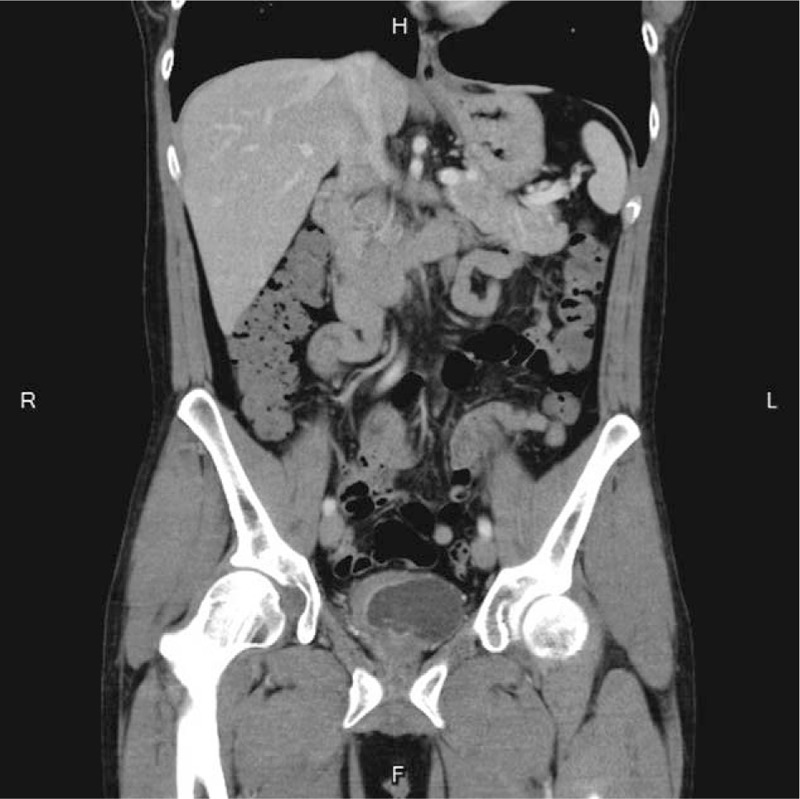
CT showed asymmetrical wall thickening (thickness of up to 1.2 cm) of the anterior aspect of the urinary bladder with mural nodules and mucosal enhancement with perivesical fatty stranding.
